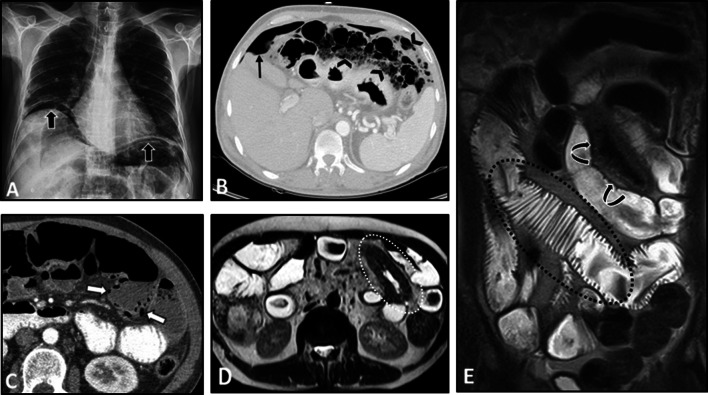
Intestinal vasculitis and benign intestinal pneumatosis in a 52-year-old male with a history of known Scleroderma presenting with abdominal pain. Physical examination revealed generalized abdominal tenderness without rebound or guarding. Upright CXR (A) was done in the ER. Pneumoperitoneum (thick black arrows) was noted at CXR in the absence of signs or symptoms of peritonitis. Due to this discrepancy, abdominal CT scan (B, C) was performed and confirmed pneumoperitoneum (thin black arrow) along with extensive intestinal pneumatosis in the transverse colon (black arrowheads) and jejunum (thick white arrows). The patient underwent conservative treatment. He was discharged one week later with improved signs/symptoms. After three weeks, MRE was obtained and displayed no pneumoperitoneum. Axial T2-W image (D) demonstrates segmental jejunal mural thickening (white dotted oval) with hypointense appearance. Coronal T2-W image (E) shows bowel dilation and the classic hidebound sign (black dotted oval) in the ileum related to known scleroderma. The T2 hypointense segment of thick jejunum is also seen (curved arrows), suggestive of chronic mural thickening perhaps due to fibrosis
